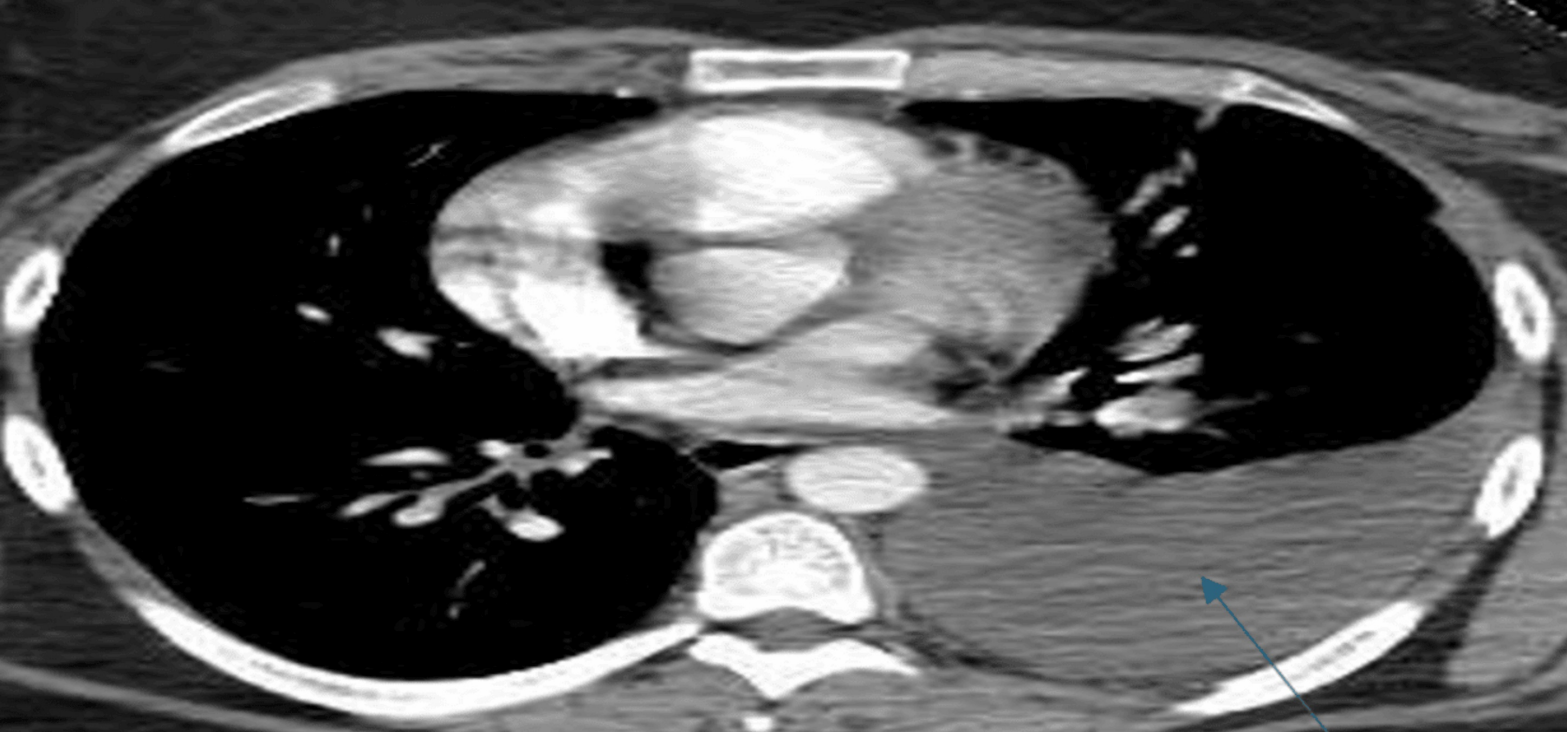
Arrow pointing to previous CT scan done three weeks ago with left pleural effusion
